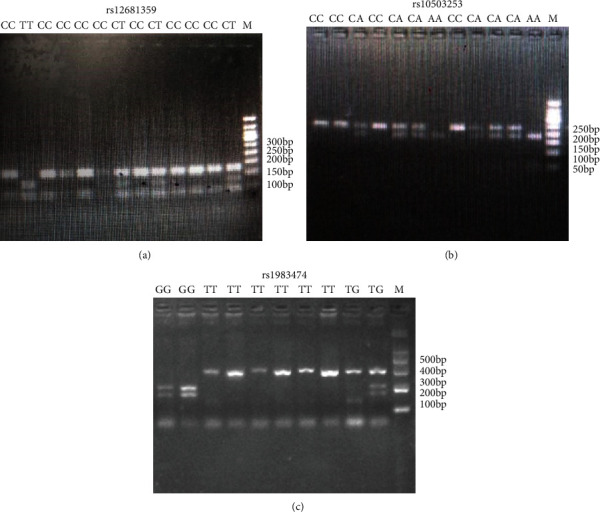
Polymorphism for rs12681349 (CC/CT/TT), rs10503253 (CC/CA/AA), and rs1983474 (GG/TG/TT) was genotyped by PCR-RFLP.
